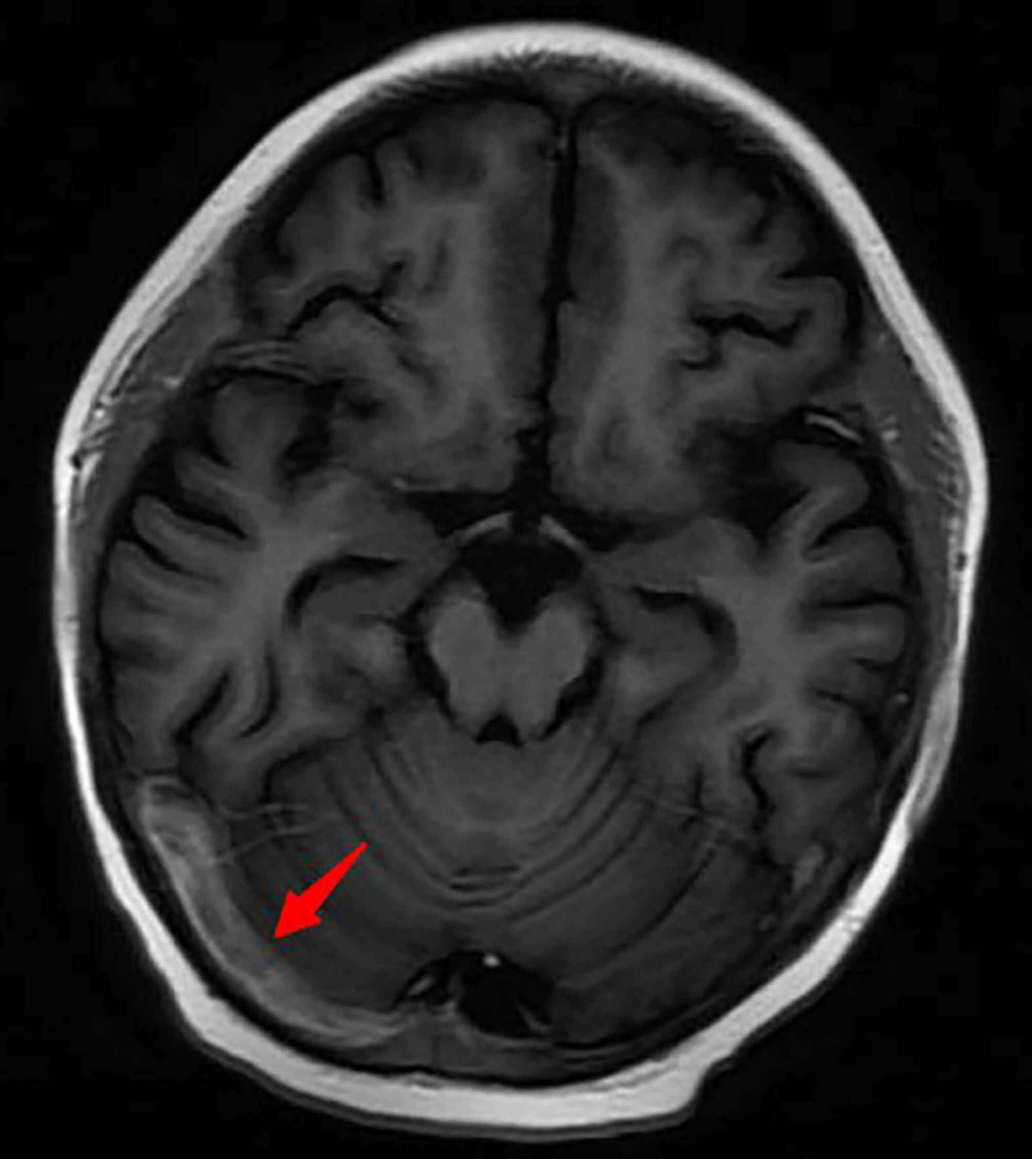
Brain MRI On day 17, 3D MRV shows that the thromboses are less severe than before.
